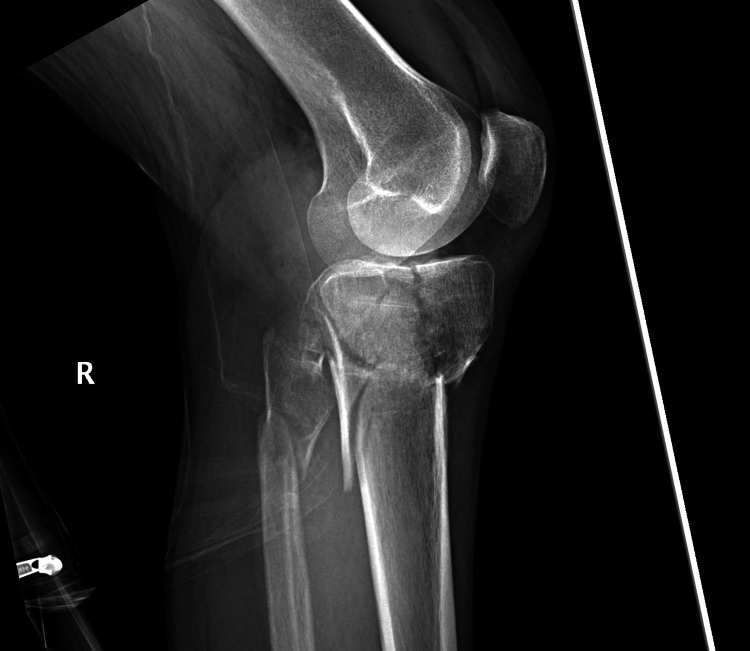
Lateral radiograph of the injured limb. There is minimal displacement on the lateral view of the knee. Mildly displaced fibula fracture is also visible.
